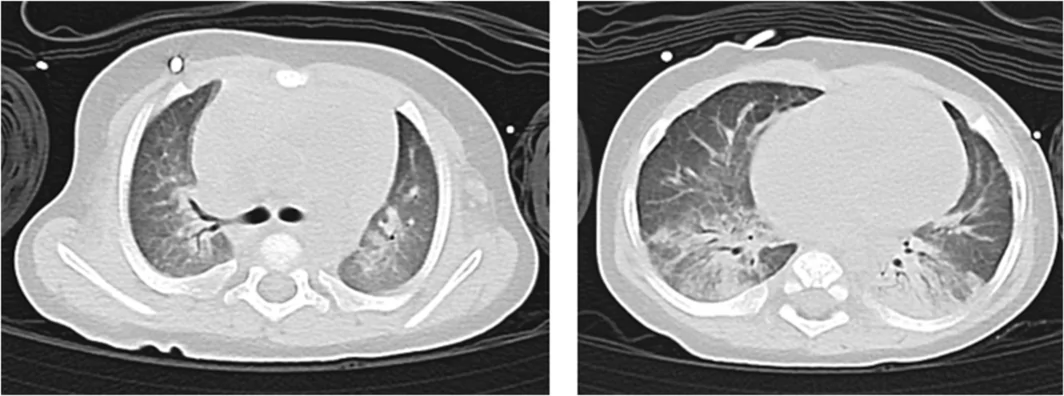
Non‐contrast chest computed tomography (CT) imaging. Diffuse bilateral lower lobe and central ground glass opacities with interlobular thickening and lower lobe consolidation.
